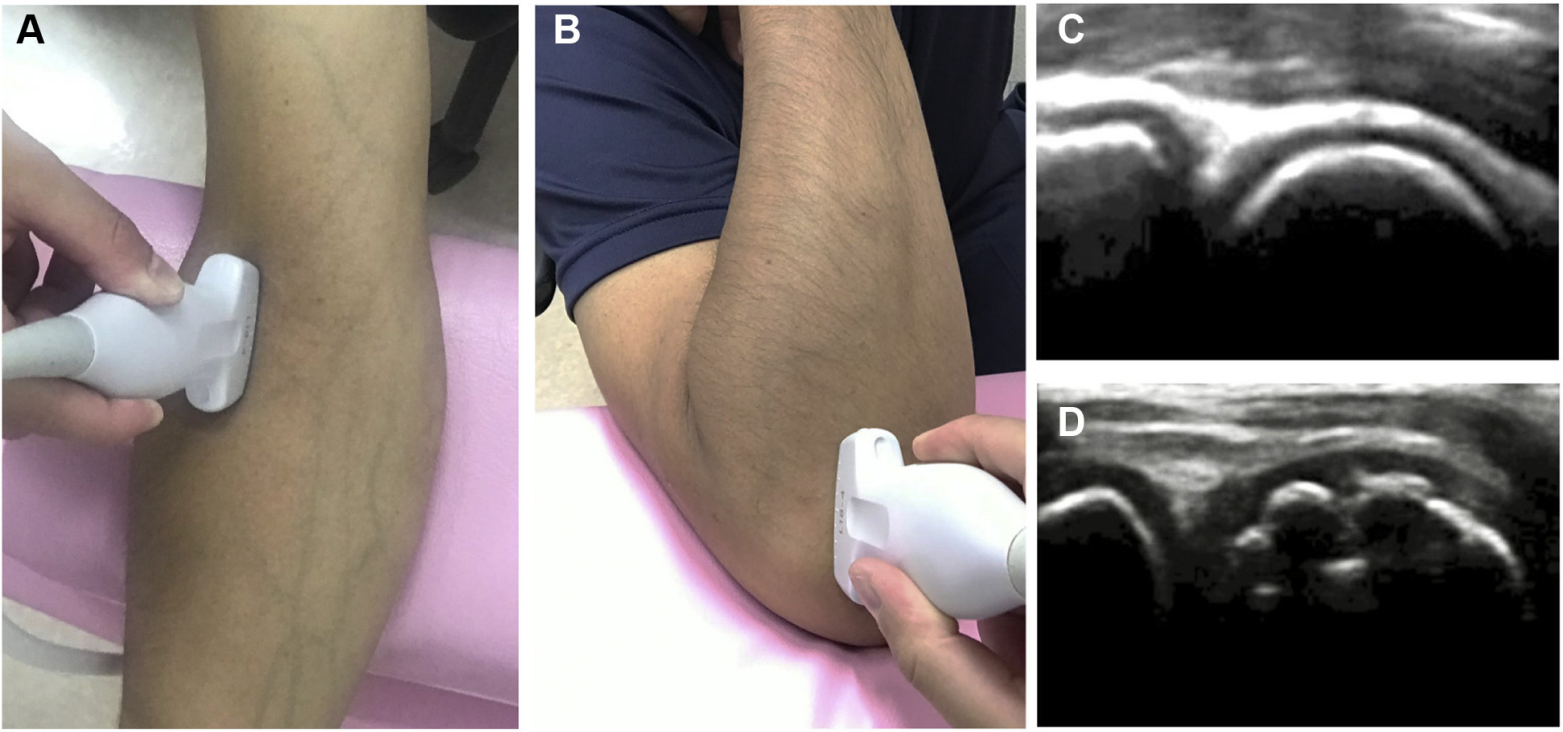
Ultrasonographic examination of the humeral capitellum. To assess the humeral capitellum, the transducer is first placed on the anterolateral aspect of the elbow in the maximally extended position (A) and then moved to the posterolateral aspect of the elbow in the maximally flexed position (B). The subchondral bone is smooth in the normal capitellum (C). Capitellar OCD is diagnosed by irregularity or fragmentation of the subchondral bone of the capitellum (D). *OCD*, osteochondritis dissecans.
